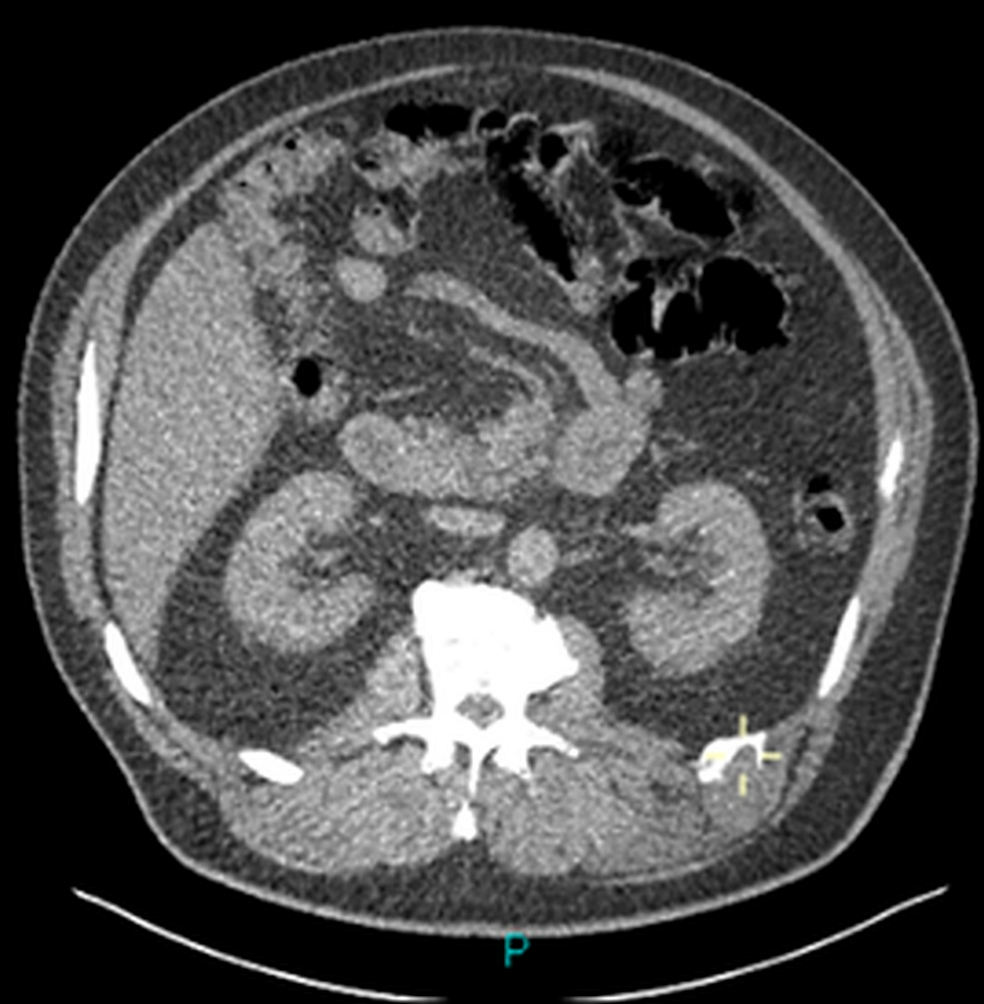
CT scan axial view. CT showing osteolytic lesion in the left 12th rib with the remodeling of the adjacent bone and a lobulated periosteal pattern with mild compression of the adjacent muscles.
